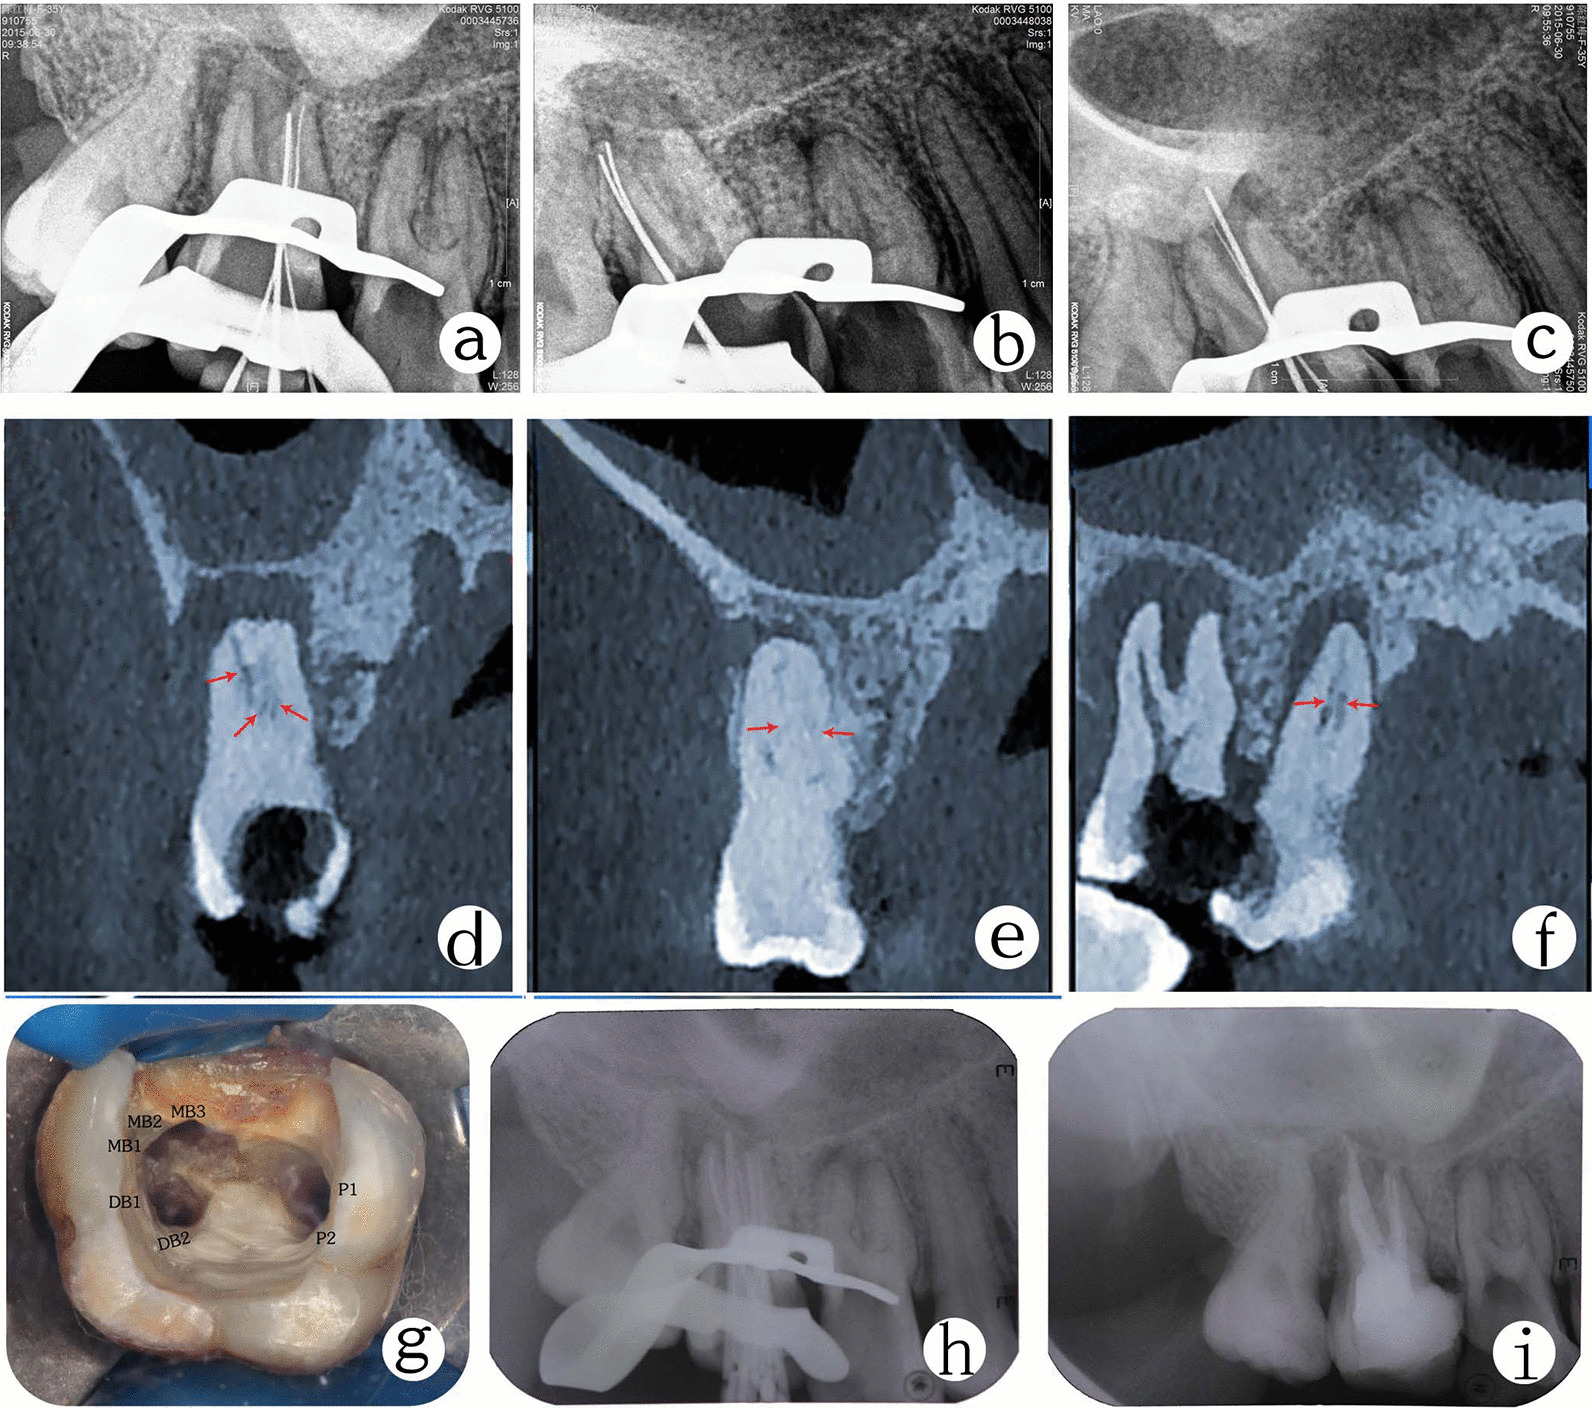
Working length radiograph of tooth #17 in the a mesiobuccal, b distobuccal and c palatal roots. The MPR views of CBCT showing canal configuration in the d mesiobuccal root canal, e distobuccal root canal, and f palatal root canal. g Root canals orifices. h Master cone fit. i Post-obturation radiograph
